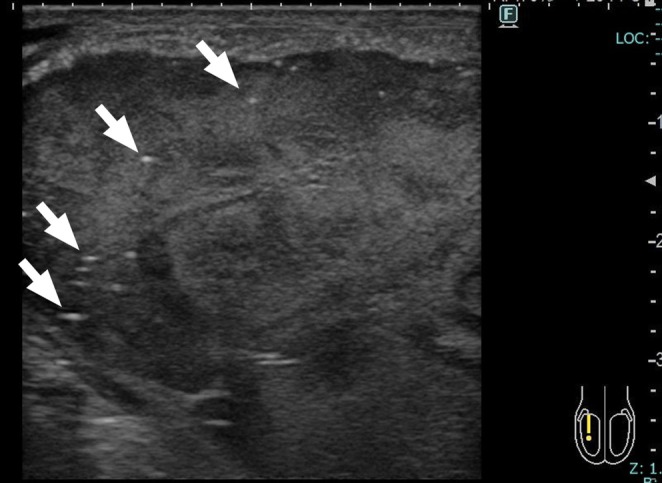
Ultrasonographic findings of the right testis. The tumor occupies most of the testis. The tumor shows heterogeneous echogenicity and contains numerous hyperechoic foci (arrows).
